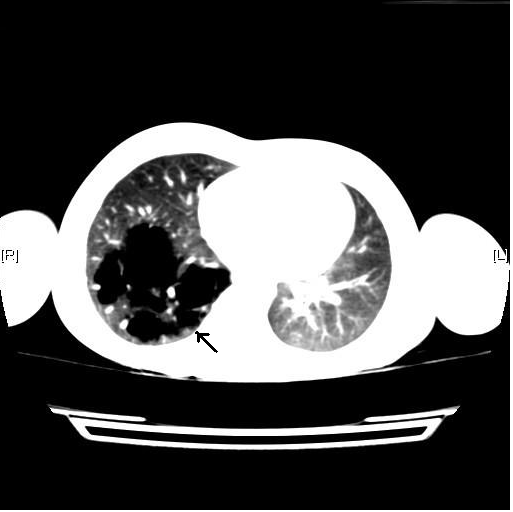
Computed tomography scan of the chest showing multiple cystic lesions of congenital pulmonary adenomatoid malformation in the right lower lobe (arrow).
